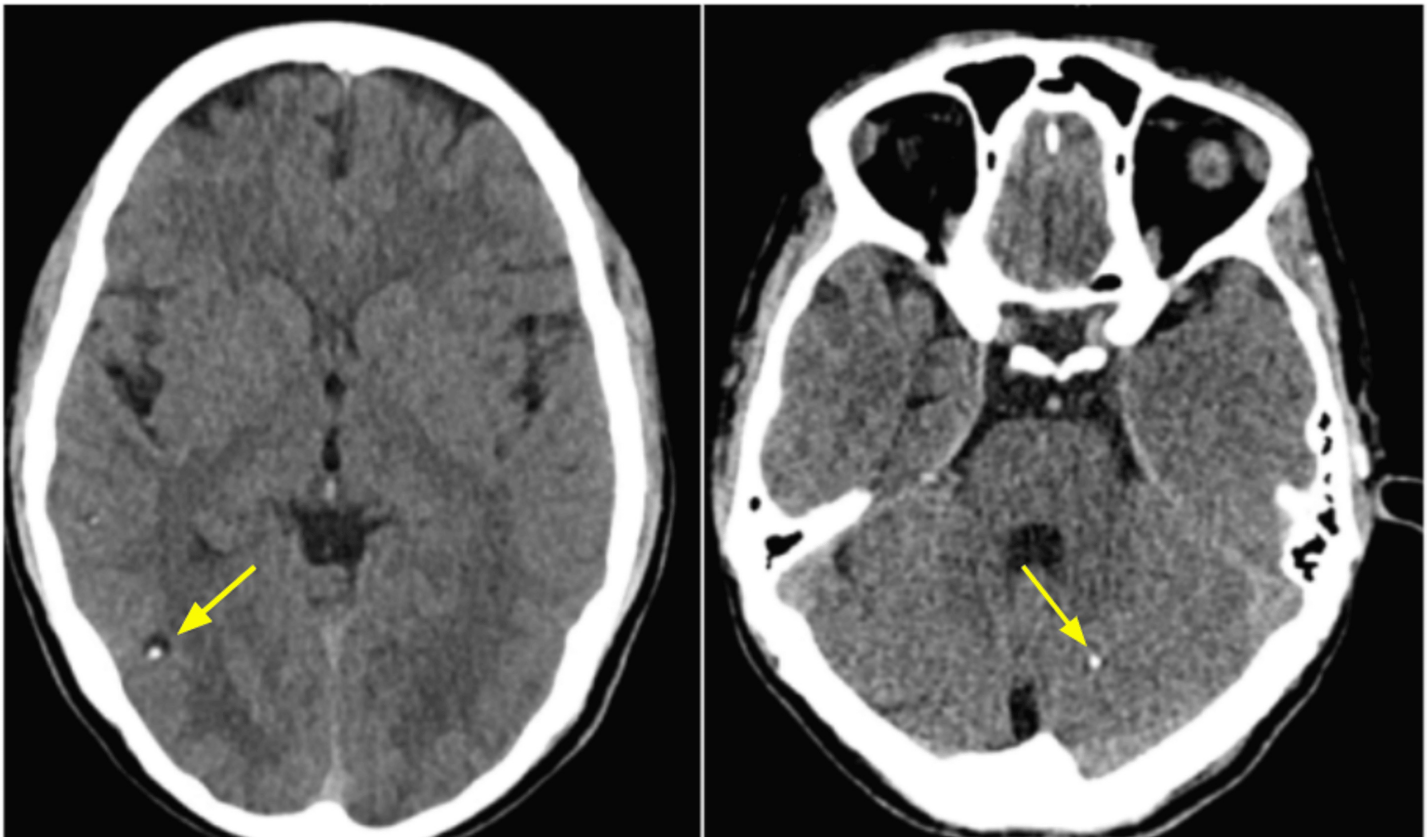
Brain CT imaging findings in a patient with multiple parenchymal neurocysticercosis, showing a vesicular cyst in the right posterior lateral parietal lobe (left), measuring up to 12 mm, and a calcified cyst in the left cerebellum (right).
